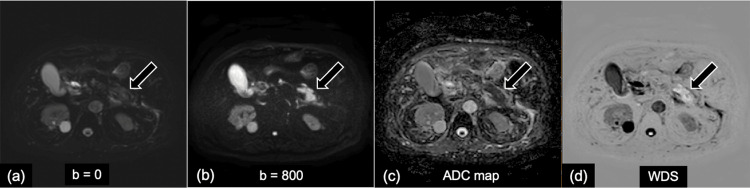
Representative case of a man in his 70s with focal-type autoimmune pancreatitis (AIP) in pancreatic tail. (a) diffusion-weighted image (DWI) b value = 0 s/mm^2^, (b) DWI b value = 800 s/mm^2^, (c) apparent diffusion coefficient (ADC) map, (d) weighted diffusion subtraction (WDS) image. (a, b) On the DWI (b = 0, 800 s/mm^2^), an irregularly shaped mass located in the tail of the pancreas shows high signal intensity compared with the normal pancreas parenchyma. (d) The WDS image shows the mass with high-signal-intensity "white-out."
